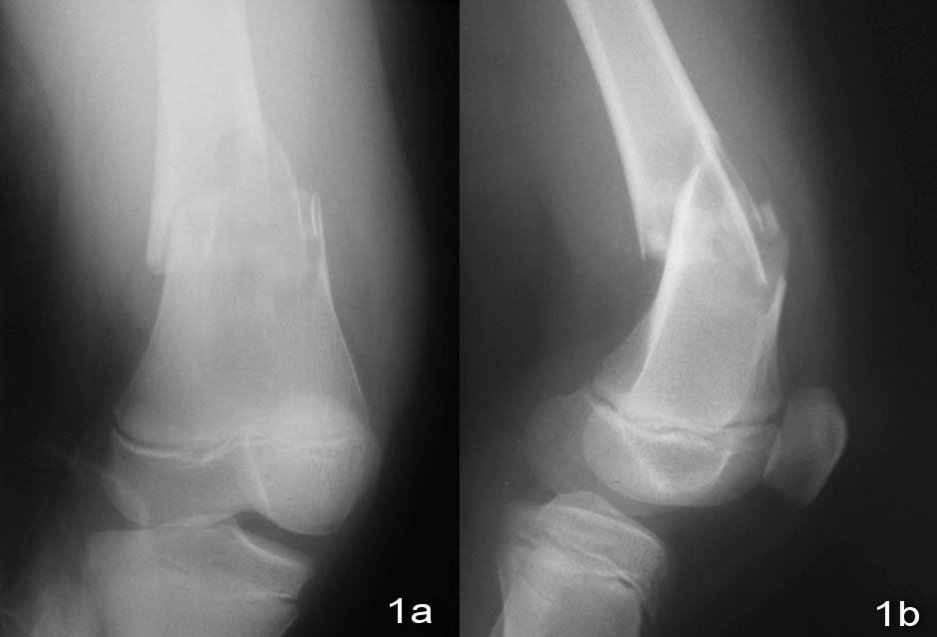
X-ray of right knee showed comminuted supracondylar fracture of femur.
